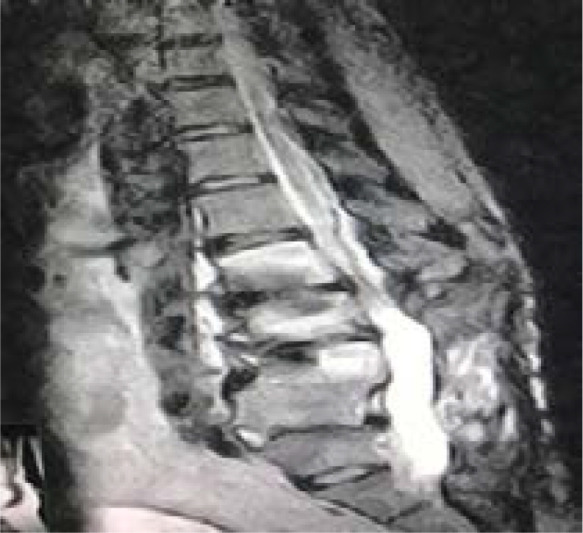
Metal induced artifact seen on lumbar spine MRI on patient with known inherent metallic implant
